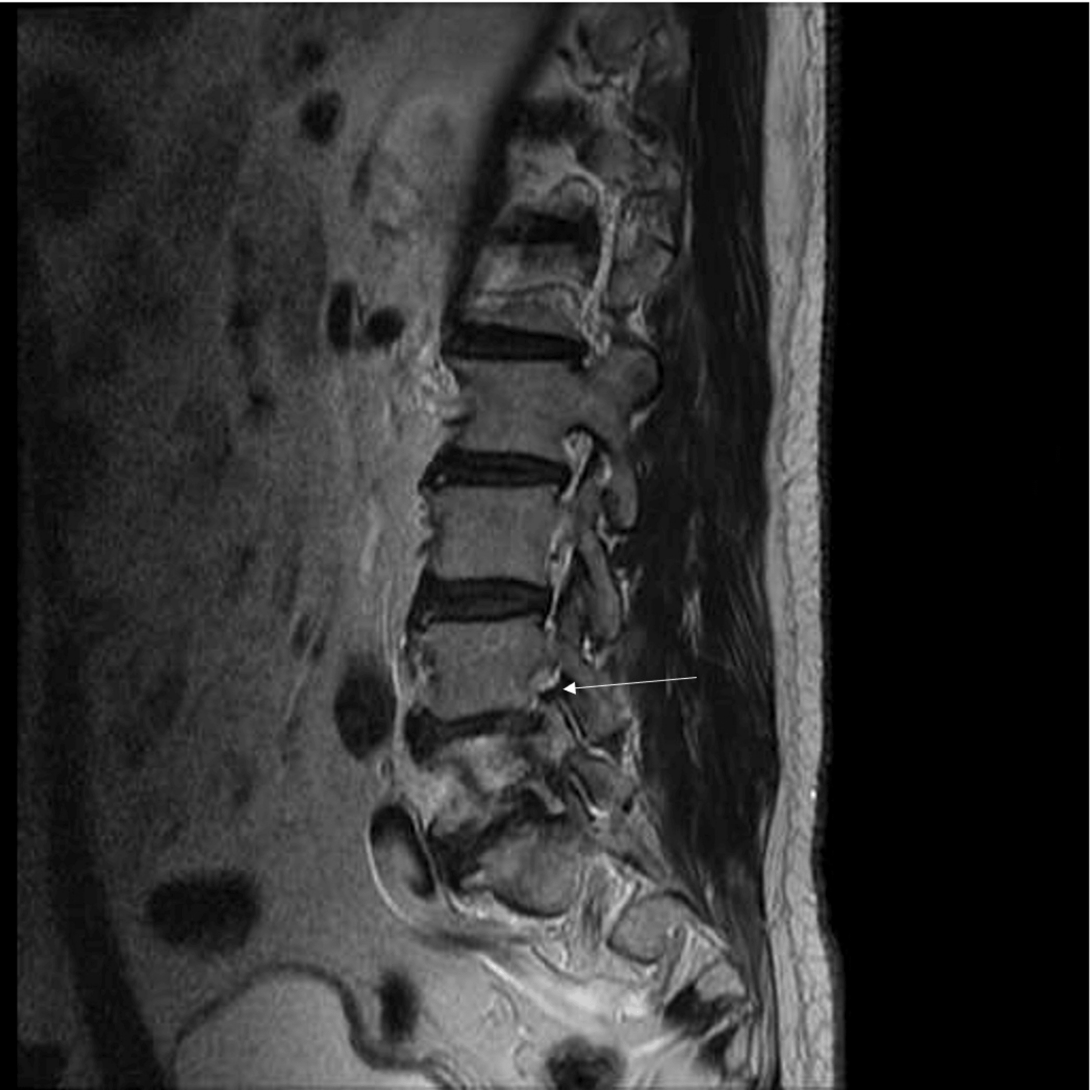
Preoperative lumbar MRI results on sagittal T2-weighted images MRI: magnetic resonance imaging Lumbar MRI demonstrates significant left-sided L5 foraminal stenosis (white arrow)
